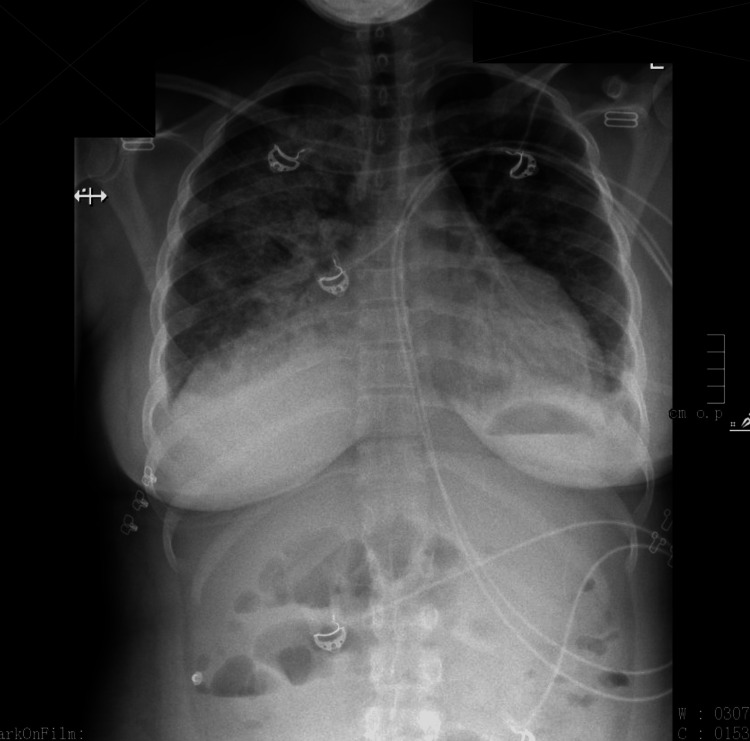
Chest X-ray showing bilateral pleural effusions (right > left) and right-sided perihilar infiltrate with pulmonary opacities The findings are compatible with pulmonary edema vs. lobar pneumonia
